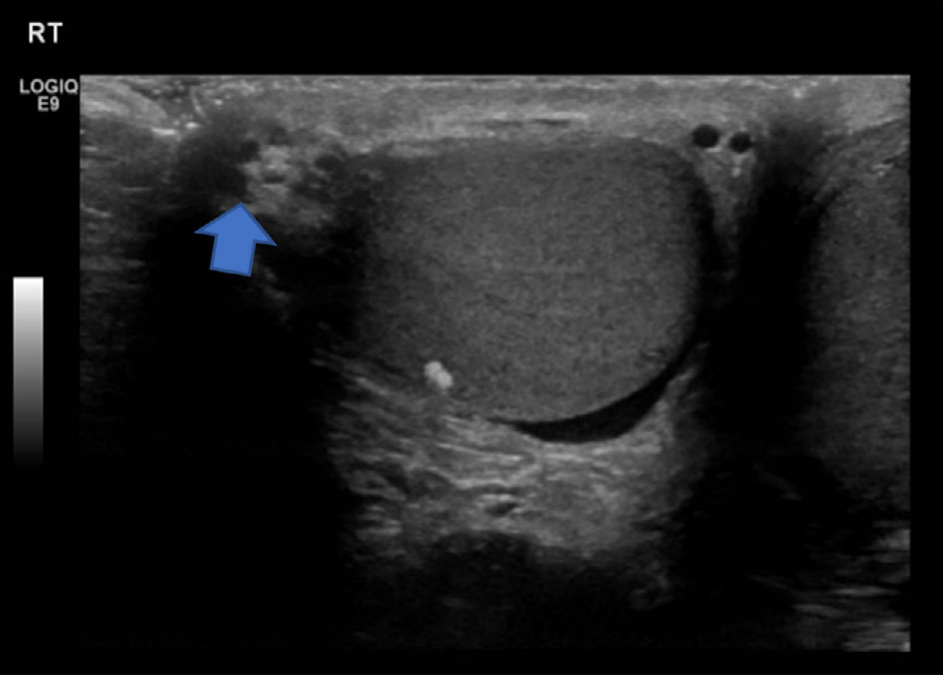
Right testicular ultrasound showing a heterogenous lesion at the superior pole of the testis (arrow).
